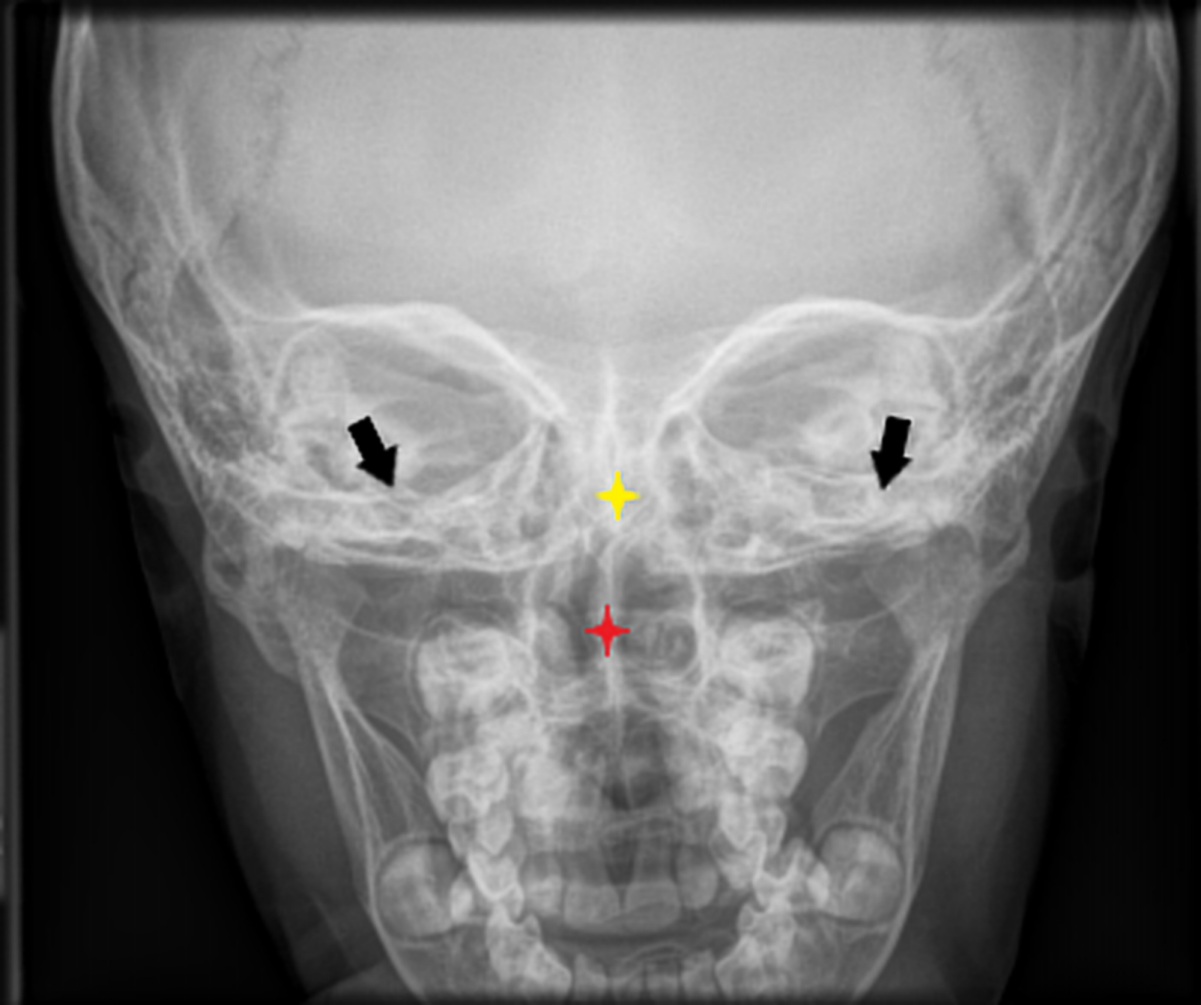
Frontal X-ray imaging of the face at age 5, which shows a slightly deviated nasal septum to the right (red star) but no structural abnormalities or fractures Yellow star = cribriform plate, red star = nasal septum, black arrows = inferior orbital plate
